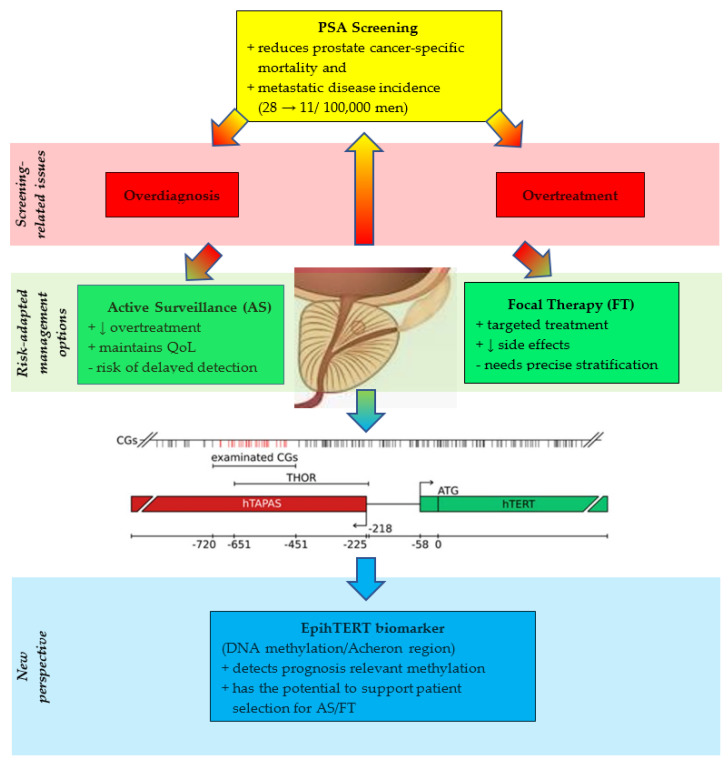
We are at the threshold of solving the PCa screening dilemma by elucidating the emerging role of EpihTERT. Overview of the proposed integration of the EpihTERT assay into contemporary prostate-cancer diagnostic pathways. Yellow, screening method. Red, screening-related issues. Green, risk-adapted management options. Blue, EpihTERT biomarker supplementing screening to reduce overdiagnosis, overtreatment and overtherapy using the exact location of the examined CpG rich region of a 215 bp 5′- fragment within THOR, ranging from −482 to −696 relative to the translational start site of *hTERT* gene as described in detail [40,42]. In men with elevated or rising PSA, liquid-biopsy EpihTERT testing may serve as a pre-biopsy triage tool to enrich for clinically significant, telomerase-active tumors. In patients undergoing MRI-targeted or systematic biopsy, tissue-based EpihTERT assessment can further refine risk stratification, particularly in the Gleason 6–7 range. Serial EpihTERT measurements may additionally support longitudinal monitoring during active surveillance or after local therapy. PSA, prostate specific antigene; QoL, quality of life.
